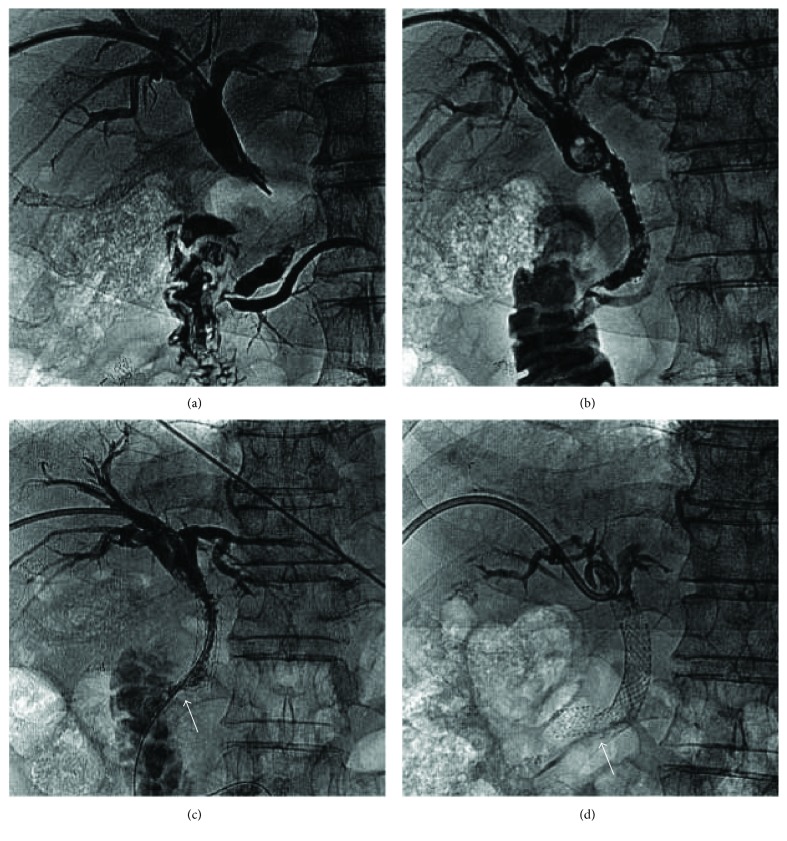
Fracture in a 71-year-old man with common bile duct obstruction secondary to cholangiocarcinoma. (a). Percutaneous transhepatic cholangiogram depicting common bile duct stricture was shown. (b). Two weeks later, a stent (diameter, 10 mm; length, 40 mm; COOK Inc., Bloomington, IN, United States) was inserted into the biliary stricture, above the duodenal ampulla. (c). Nine months after stent placement, the patient developed reobstructive jaundice, and percutaneous cholangiography revealed stent occlusion. Another biliary stent (diameter, 10 mm; length, 100 mm; COOK Inc., Bloomington, IN, United States) was deployed, extending from the duodenum to the convergence of the left and right hepatic ducts. An obtuse angle was formed between the two biliary stents (arrow). (d). One hundred and forty-two days after the second biliary stent implantation, plain X-ray showed the stent fracture located at the duodenal ampulla (arrow).
